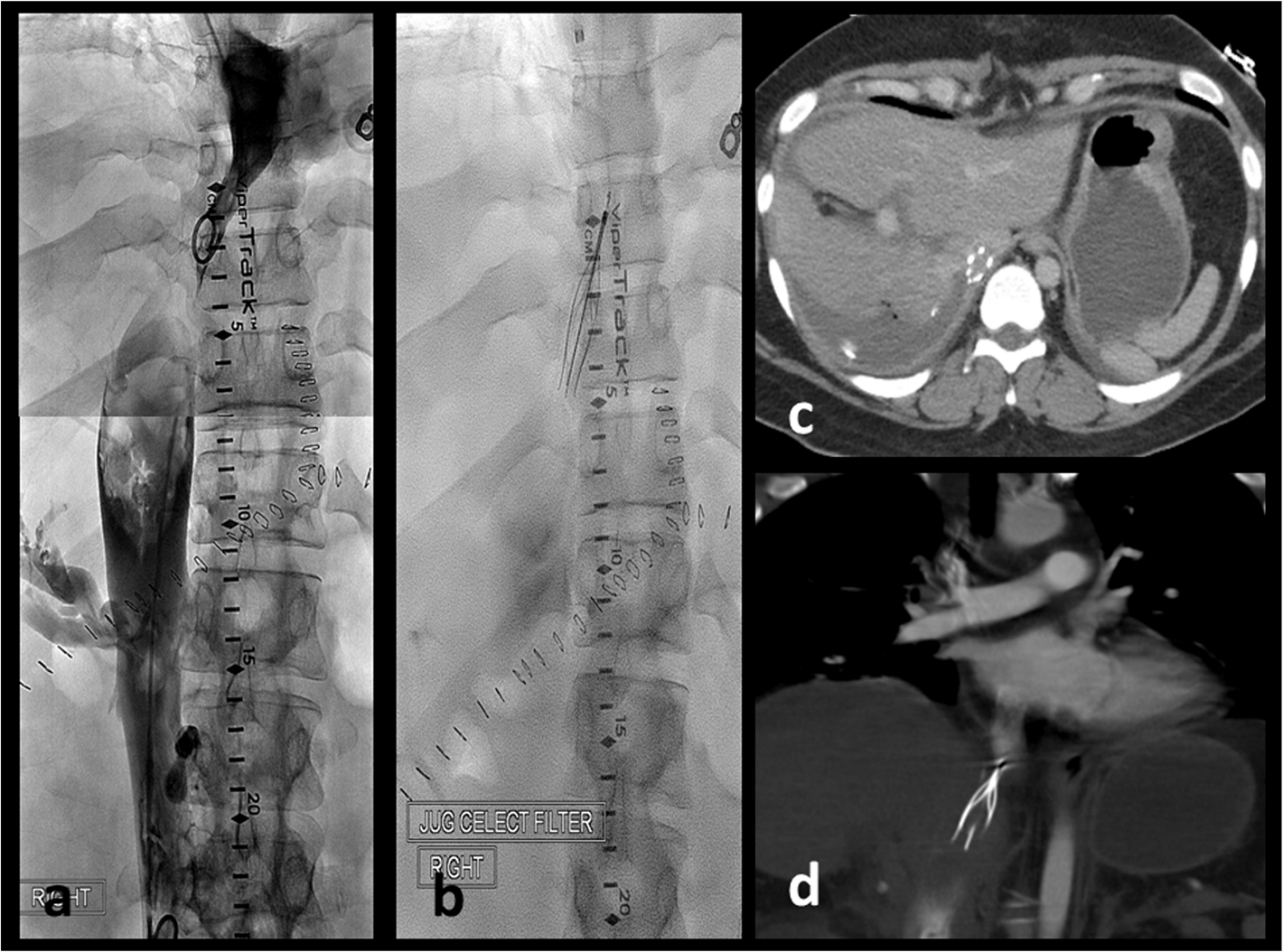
Two days following right hepatic resection, the patient developed deep vein thrombosis. a. Venogram demonstrates large clots in the suprarenal IVC and narrowing of the superior aspect of the IVC above the hepatic vein inflow. b Suprarenal IVC filter was placed superior to the thrombus. c. and d. Subsequent contrast enhanced axial CT images of the abdomen shows the liver remnant shifted towards the right into the space vacated by the right hepatic lobe. IVC was severely narrowed at the point above the hepatic vein inflow associated with thrombus in the suprarenal IVC
